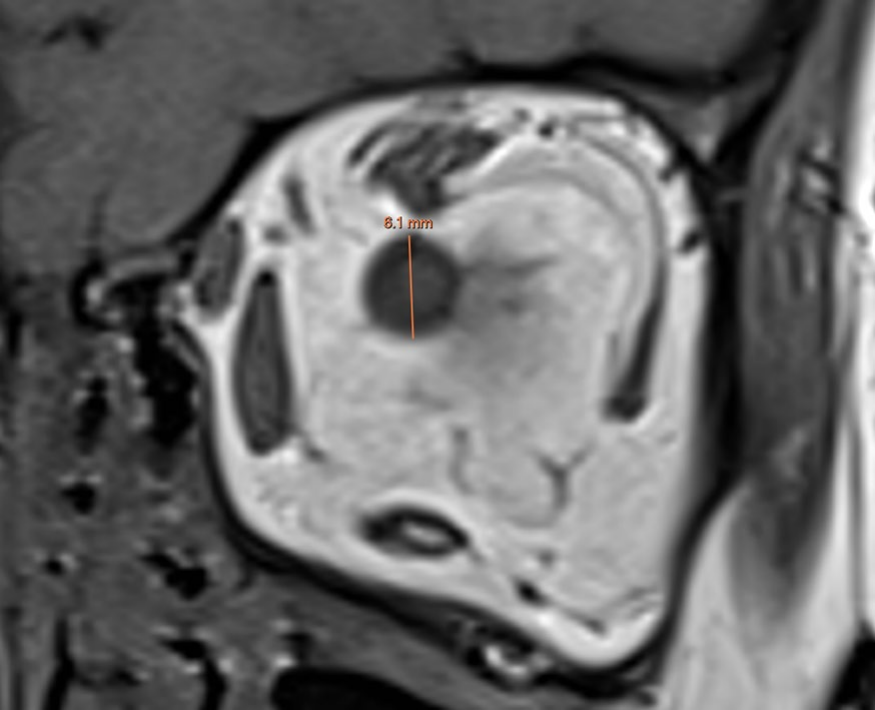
Coronal T1-weighted MRI showing the optic nerve sheath diameter measurement
